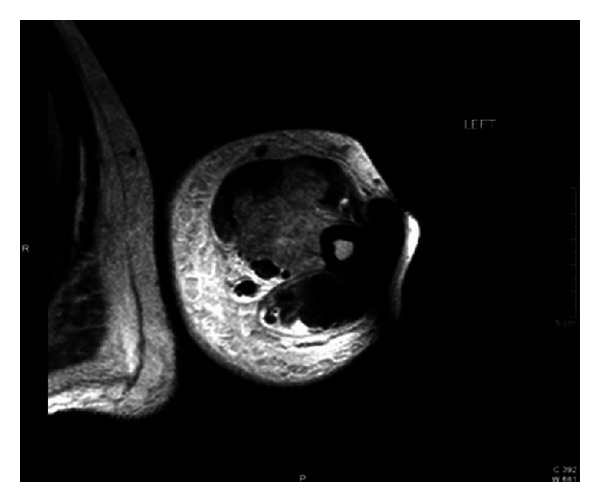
Left upper extremity MRI. T2 hyperintensity of the deep biceps, brachialis, and brachioradialis muscles in the anterior compartment.
